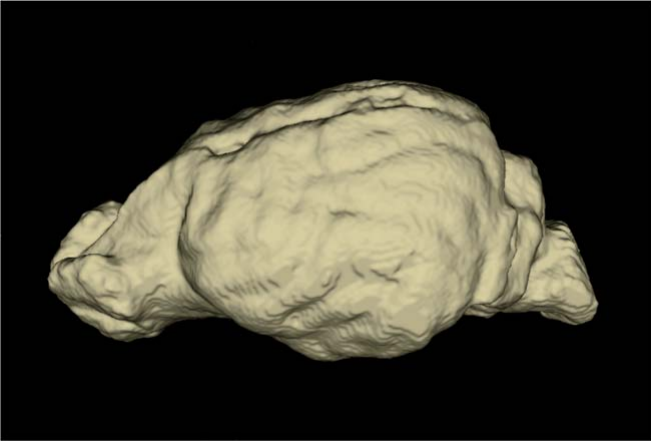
CT 3D reconstruction of *Chrysocyon brachyurus* endocast.
